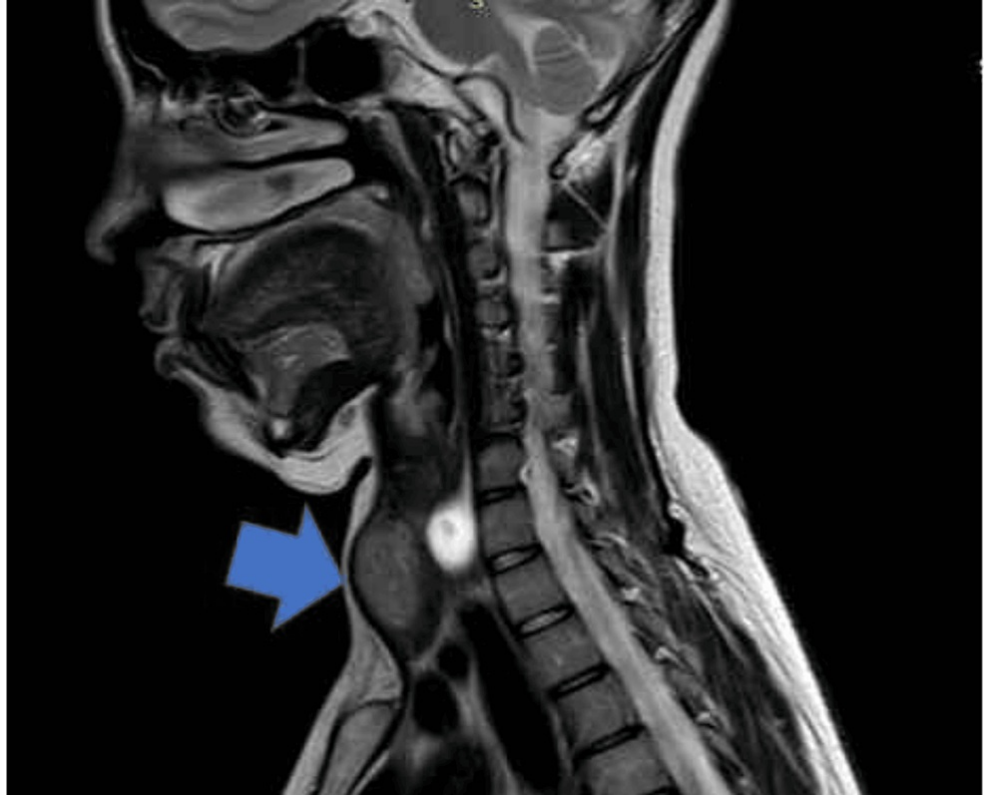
MRI neck without contrast showing thyroid mass.
